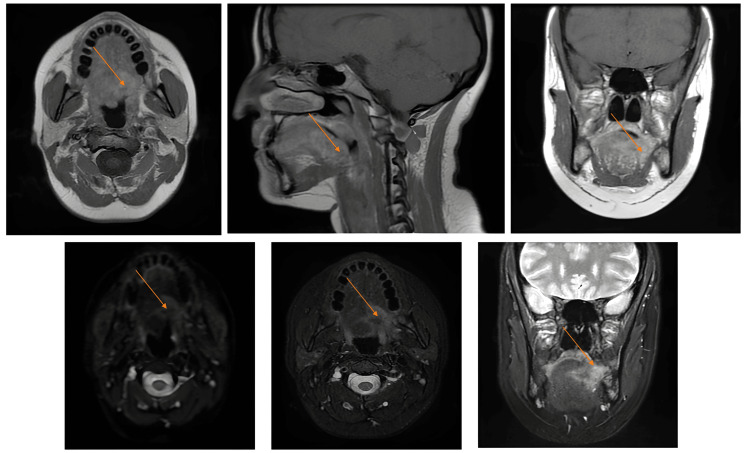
MRI images showing the process at the base of the tongue
